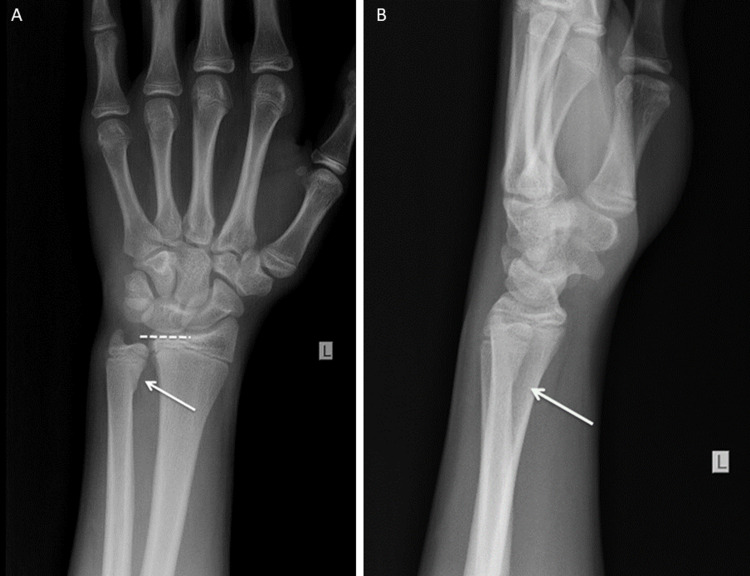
Anteroposterior (A) and lateral (B) radiographs of the injured left wrist showing the epiphyseal plate fracture (arrows).
